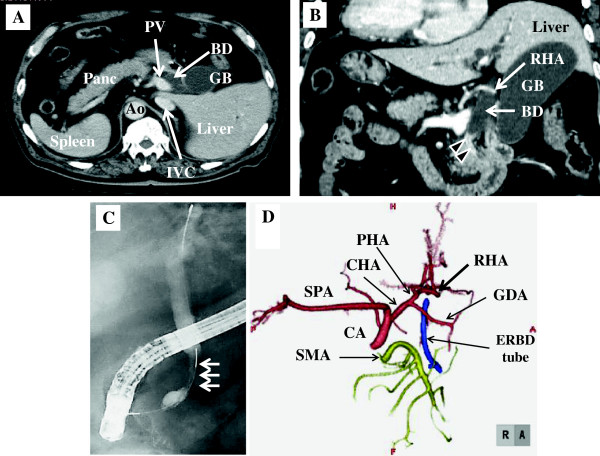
Preoperative imaging of patient 1. (A) Contrast-enhanced CT scan and (B) coronal image of multidetector-row CT reveal a dilated bile duct. (C) Endoscopic retrograde cholangiography shows distal bile duct stenosis (arrows) and upstream dilation of the bile duct. (D) Three-dimensional angiography with multidetector-row CT shows a left-to-right reversal of the vascular arrangement, but does not show vascular malformation. Ao, aorta; BD, bile duct; CA, celiac artery; CHA, common hepatic artery; ERBD tube, endoscopic retrograde bile duct drainage tube; GB, gallbladder; GDA, gastroduodenal artery; IVC, inferior vena cava; Panc, pancreas; PHA, proper hepatic artery; PV, portal vein; SMA, superior mesenteric artery; SPA, splenic artery.
